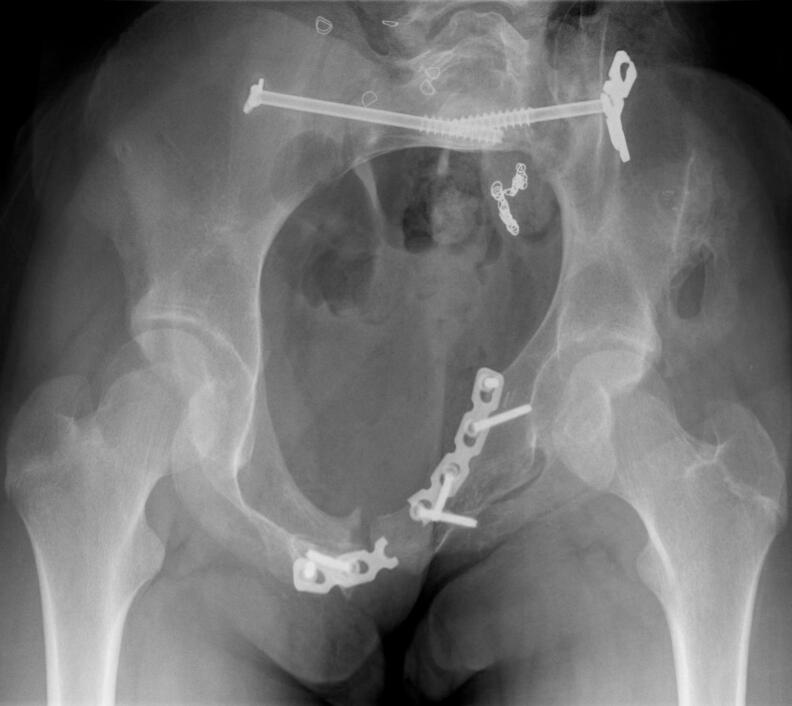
Inlet Pelvic radiograph 2.5 years after surgical fixation.
